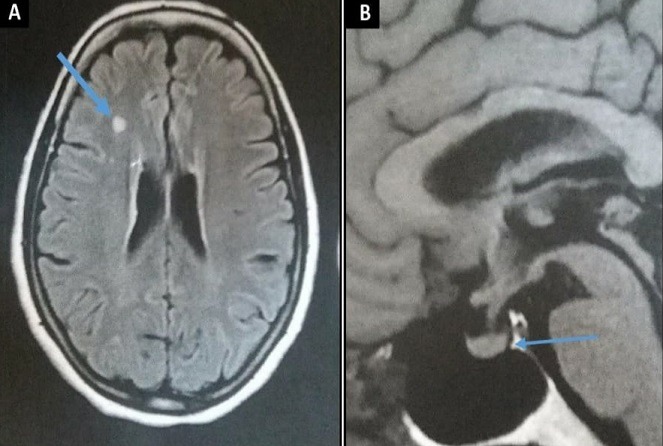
A) brain and Hypothalamo-pituitary MRI showing white matter nodular hyperintensities; B) the loss of hyperintense signal on sagittal T1 weighted image
